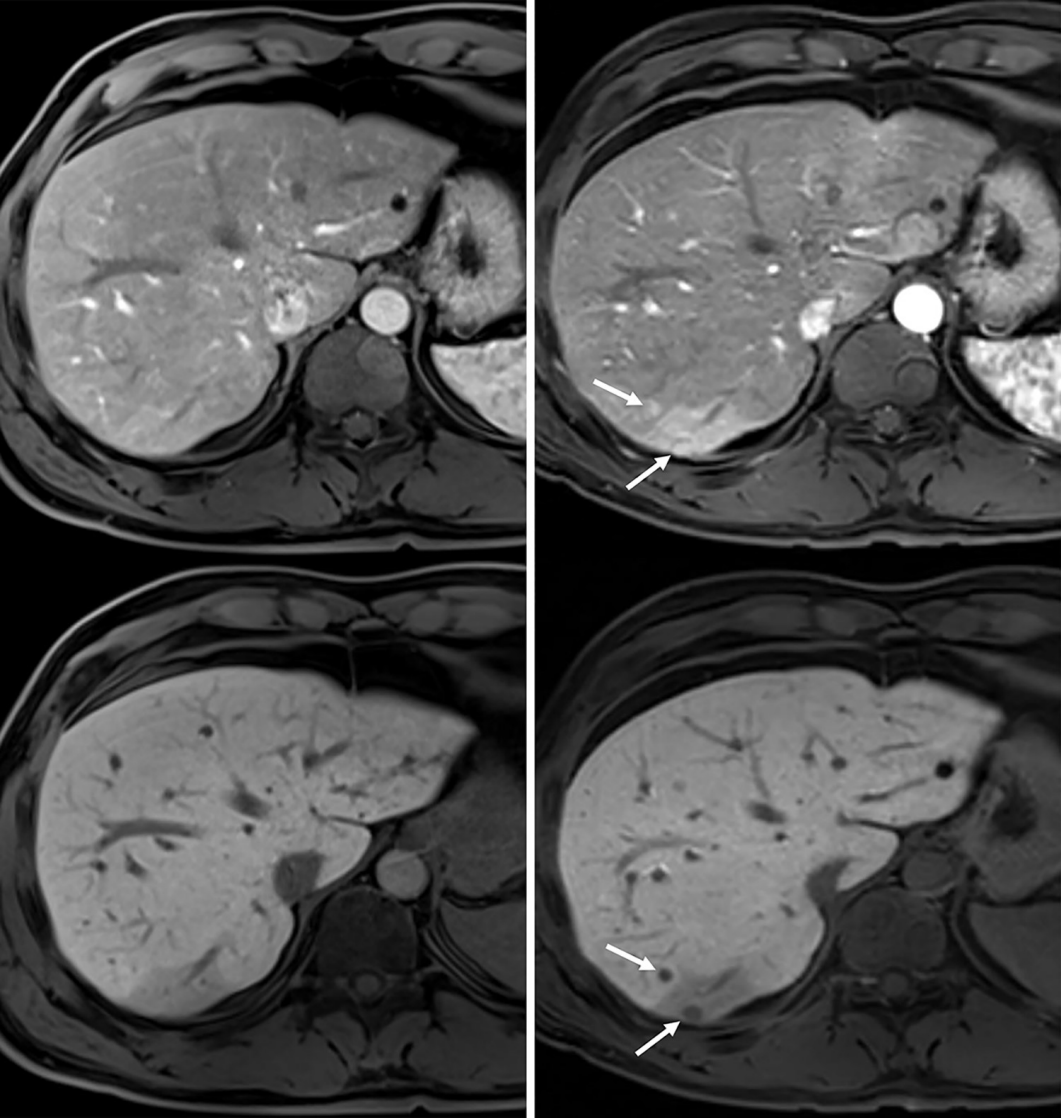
Gadoxetic acid-enhanced MRI arterial phase (upper) and hepatobiliary phase (lower) images of a 41-year-old man who had undergone liver S6 segmentectomy for hepatocellular carcinoma (HCC). (a) MRI performed 7.6 months after surgery revealed no evidence of HCC recurrence (AFP: 15.1 ng/ml; PIVKA-II: 15 mAU/ml). (b) MRI obtained after an additional 48 days revealed two new subcentimeter-sized nodules (arrows) showing arterial phase enhancement and decreased hepatobiliary phase signal intensity, indicating HCC recurrence (AFP: 119.5 ng/ml; PIVKA-II: 26 mAU/ml).
